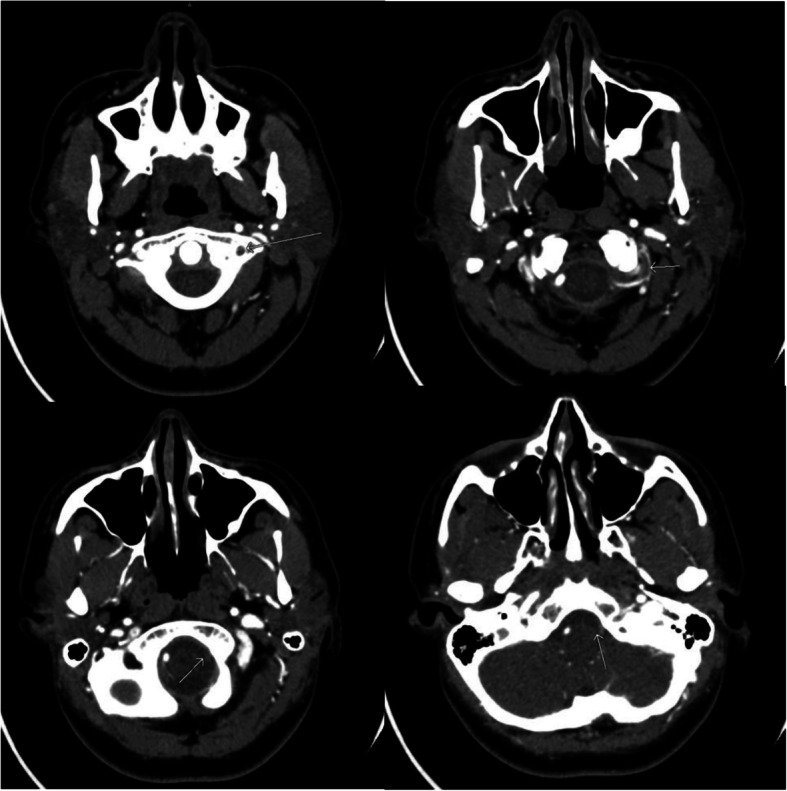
CT angiography of the head and neck demonstrates a left vertebral artery dissection originating at the C4 vertebral body level extending into the intracranial vertebral artery and involving the left posterior inferior cerebellar artery (PICA)
